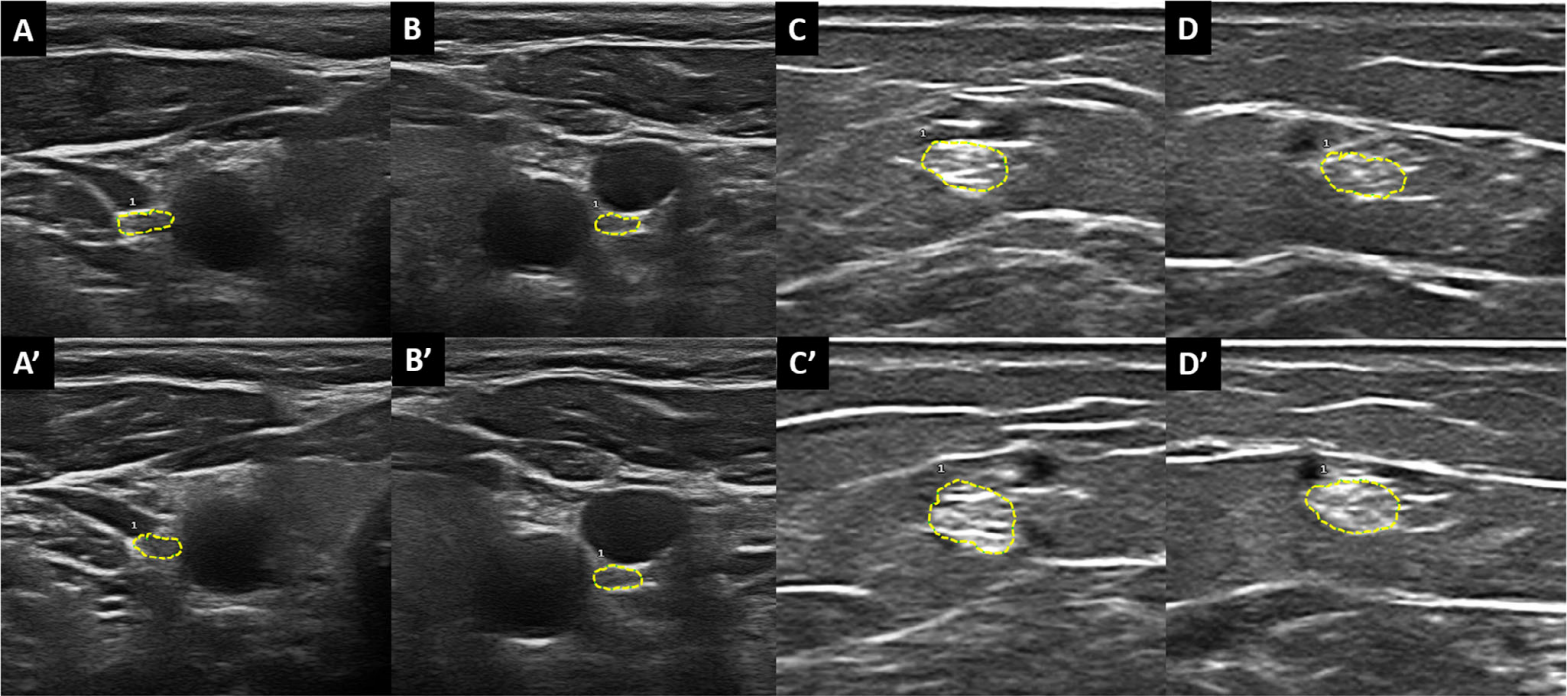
Ultrasound scans of nerves in a patient with fibromyalgia, assessed at baseline (A, B, C, and D) and at the 24‐month follow‐up (A’, B’, C’, and D’). Panels A and A’ depict the right vagus nerve, B and B’ the left vagus nerve, C and C’ the right sural nerve, and D and D’ the left sural nerve. Compared to a healthy subject, all cross‐sectional areas (CSA) are increased, measuring 5 mm^2^ for all vagus nerve assessments at both baseline and follow‐up, 8 squared millimeters (mm^2^) for the right sural nerve at both time points, 6 mm^2^ for the left sural nerve at baseline, and 7 mm^2^ at follow‐up. The CSA regions are delineated with fine yellow dashed lines.
